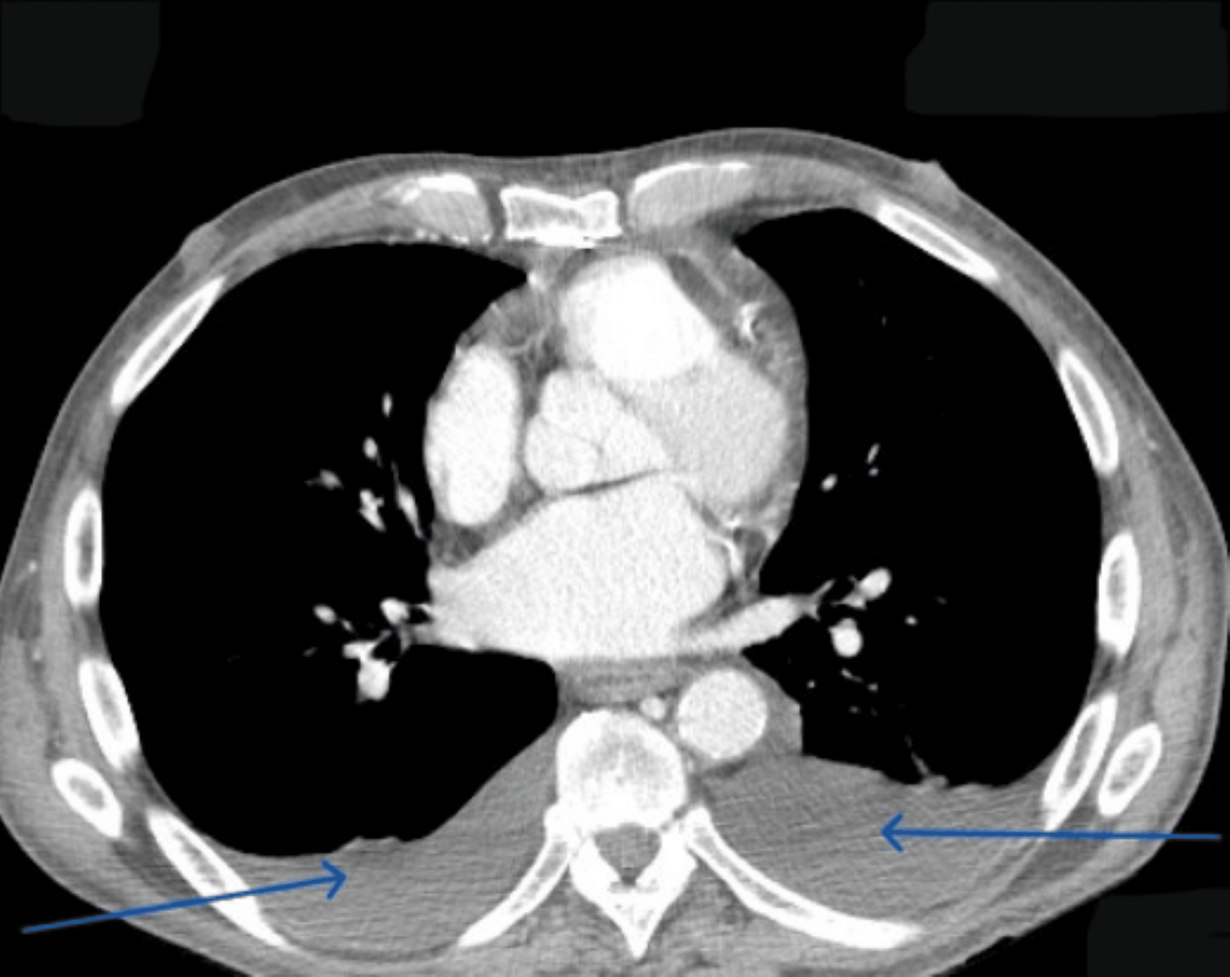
CT chest with bilateral pleural effusion (blue arrows).
